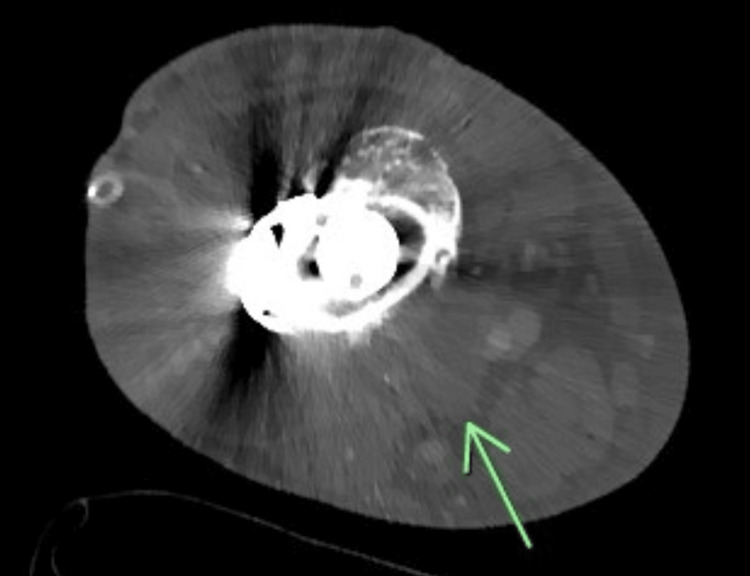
CT scan of the right lower extremity A right lower extremity CT scan with contrast revealed fluid collection with rim enhancement that was consistent with an abscess around the posterior and lateral portions of the right distal femur (Green arrow).
